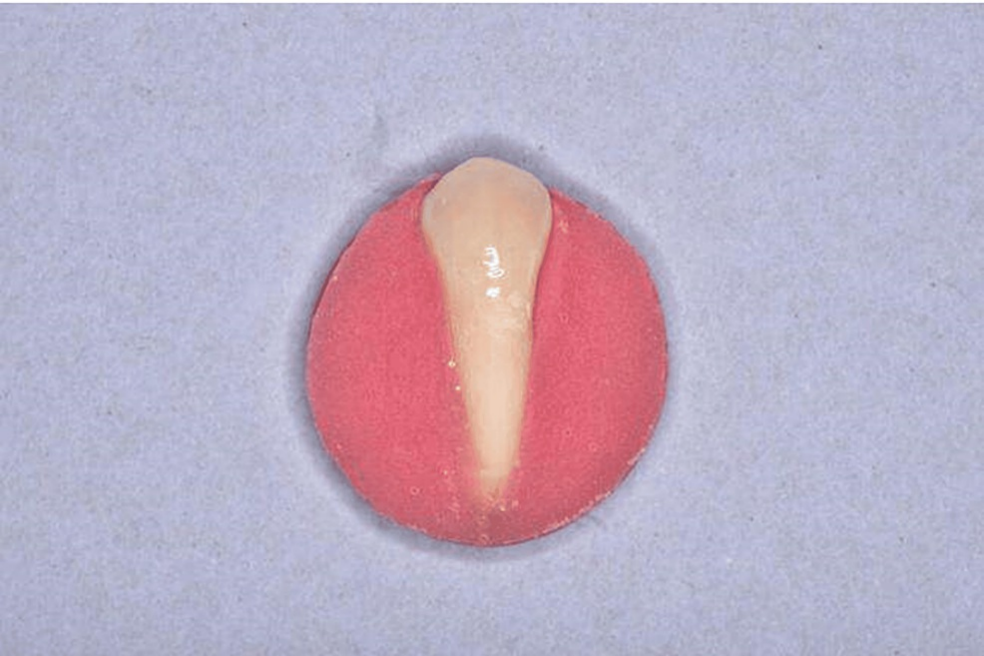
The extracted premolars were ultrasonically cleaned, dried, and mounted in resin.
